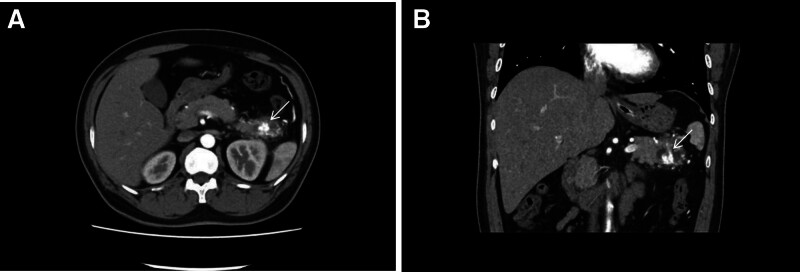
(A and B) Arterial phase-enhanced computed tomography shows a nodular hyperdense lesion in the tail of the pancreas.
